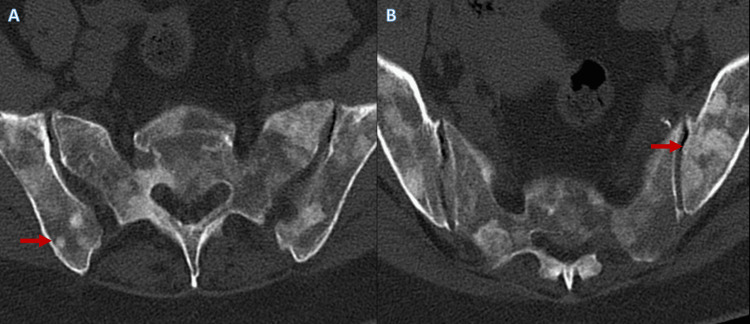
Suspicious hip bone lesions Computed tomography of the hip revealed multiple bilateral bone lesions (A and B), secondary to malignant infiltration (examples highlighted by the red arrows)
